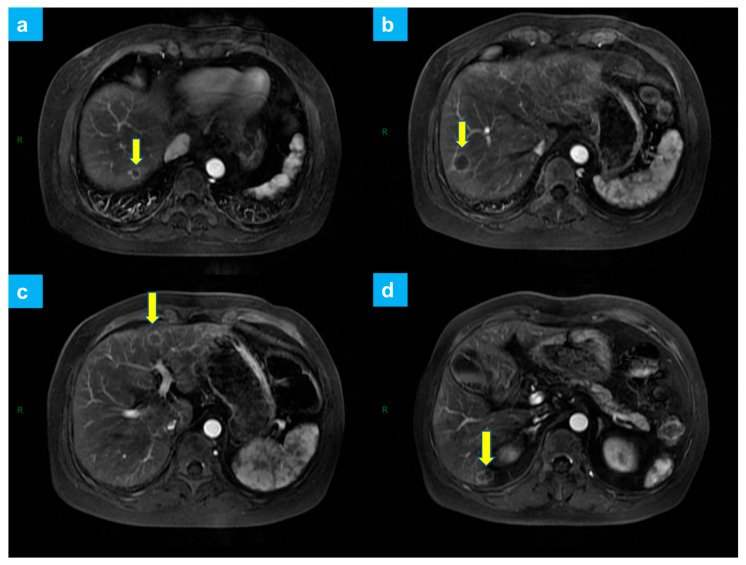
A case of TA for unresectable CRLM. Before TA, the enhanced MRI showed obvious ring-shaped enhancement in the arterial phase (yellow arrow). (a–d) show the distribution of liver metastases from four different sites in one patient.
